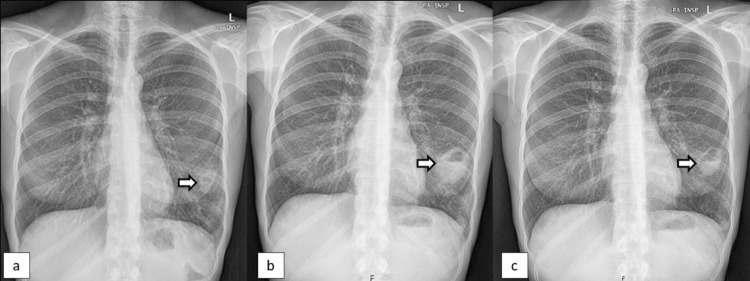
Initial chest X-rays Image (a) reveals a nodule in the left lung of the patient at the first rheumatology appointment. Image (b) shows the evolution of this nodule to a lung abscess, one month later. Image c) shows a reduction of the abscess size after three weeks of intravenous antibiotic therapy.
